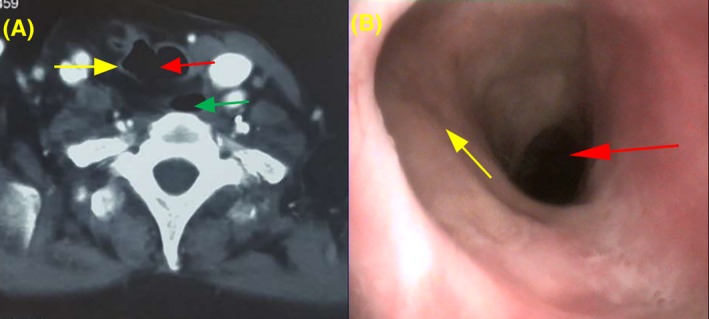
CT scan examination and electronic laryngoscopy of a trachea reconstructed with a FPTAPF. The red arrow indicates the reconstructed trachea, the yellow arrow indicates the FPTAPF, and the green arrow indicates the esophagus
